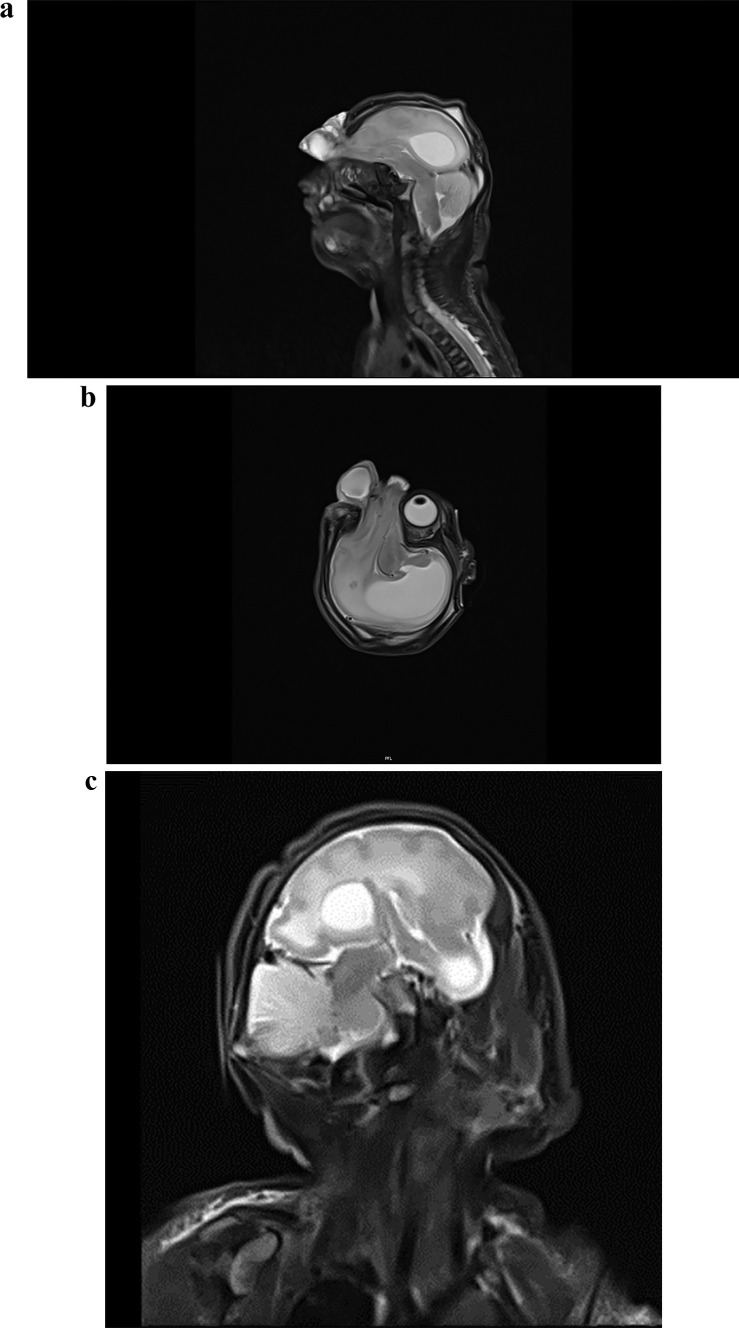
Preoperatively MRI Scans. (Sagittal, Axial and Coronal). In the T2-weighted image scans the encephalocele is identified together with the monoventricle. On the coronal slide the 90-degree hemispheric rotation is to be noted
